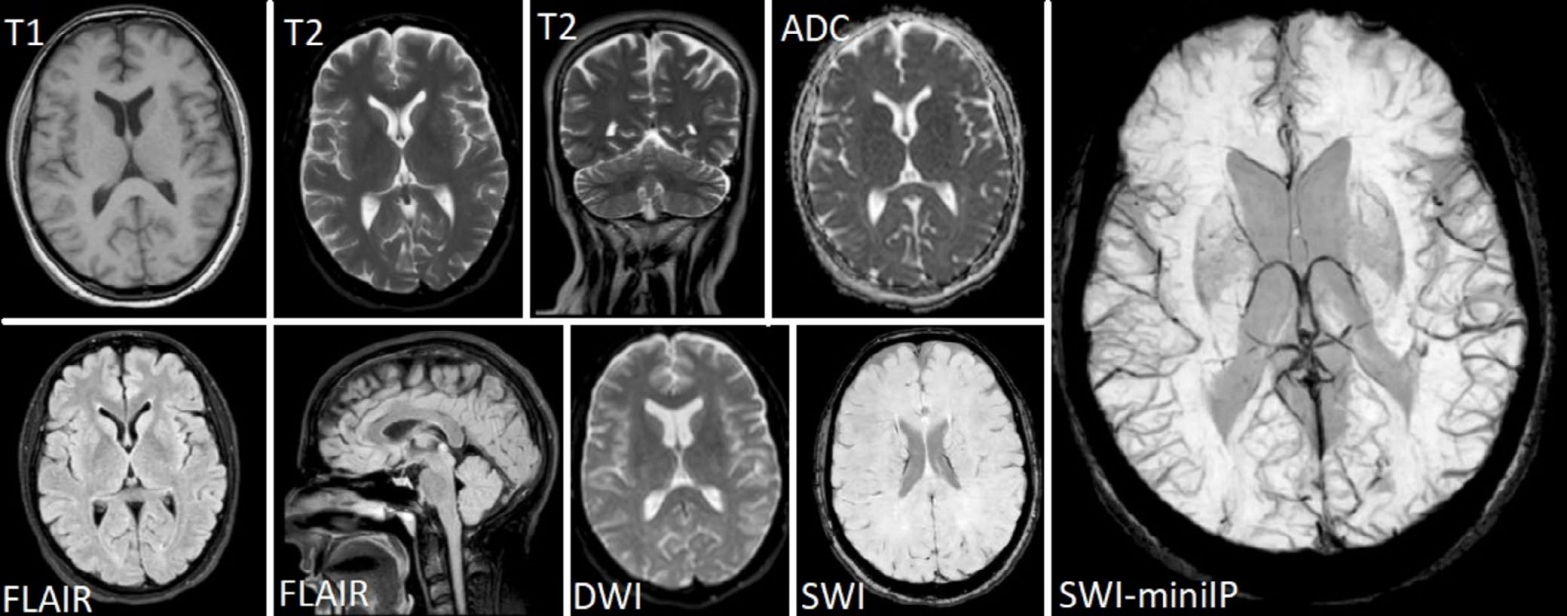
Brain magnetic resonance of the patient. The MRI of patients showed no signs of infarction, chronic hemorrhage, or ventriculomegaly. The SWI‐miniIP sequence shows no blooming artifact. ADC, apparent diffusion coefficient, DWI, diffusion‐weighted imaging, FLAIR, fluid‐attenuated inversion recovery, SWI, susceptibility‐weighted imaging.
